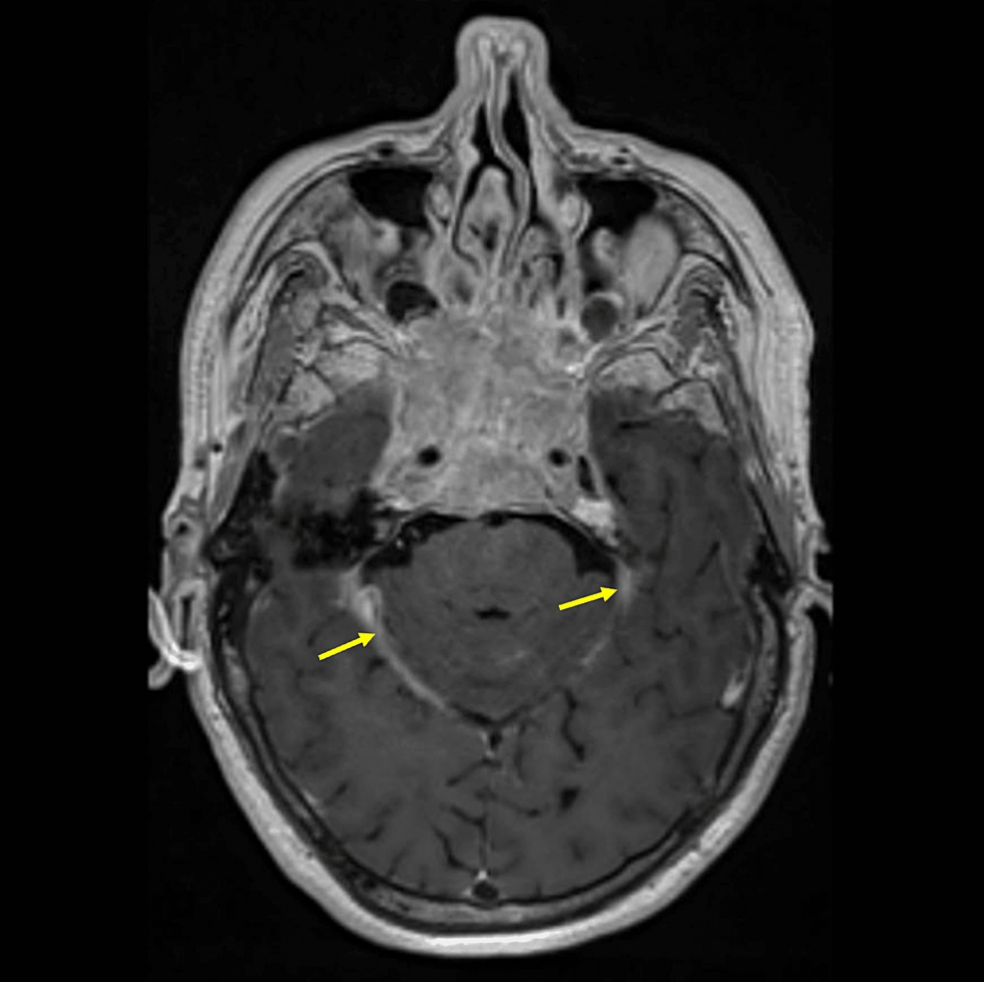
Thickening of the cerebellar tentorium on contrast-enhanced magnetic resonance imaging (indicated by yellow arrows).
